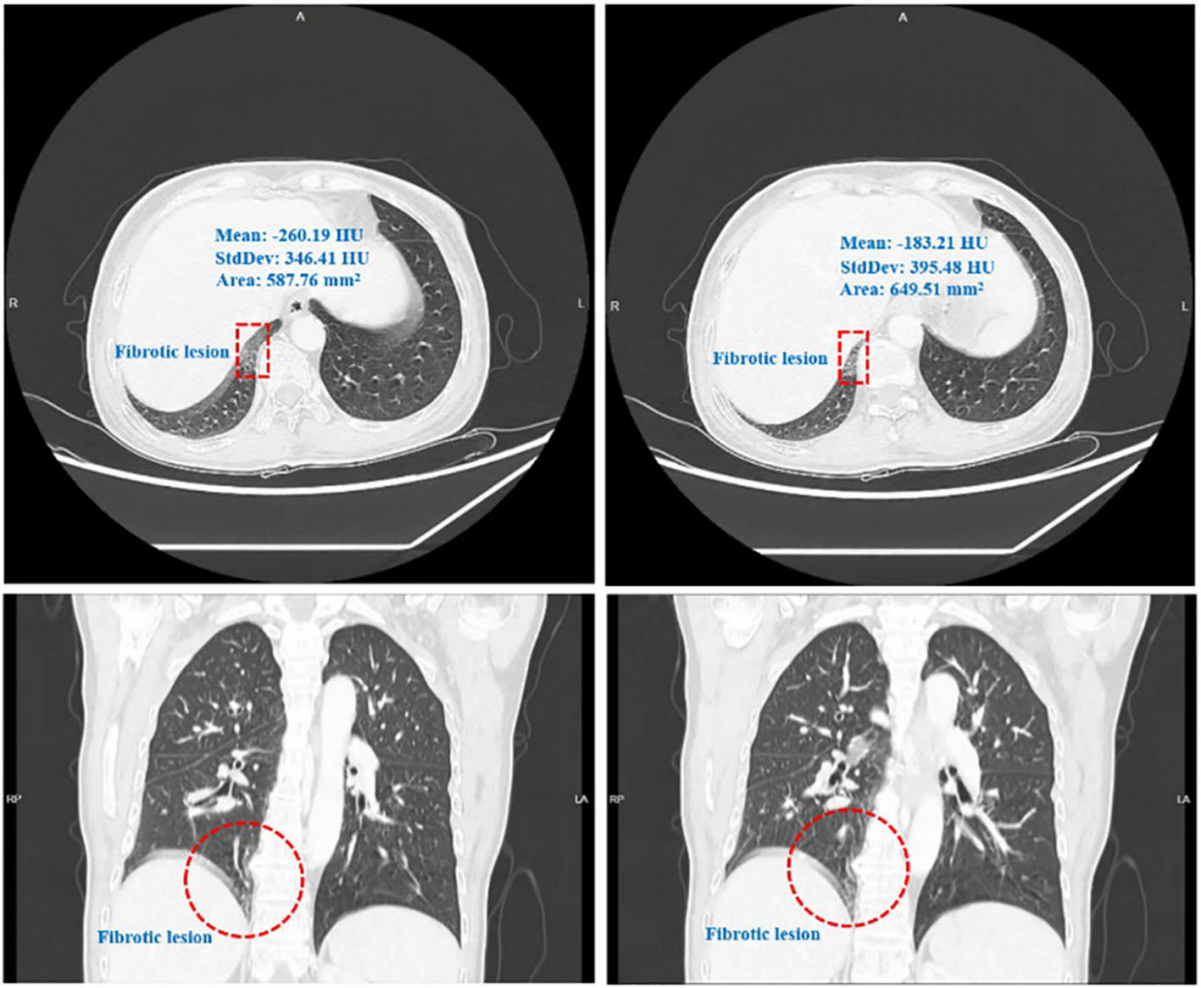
Early ILD CT imaging features.
